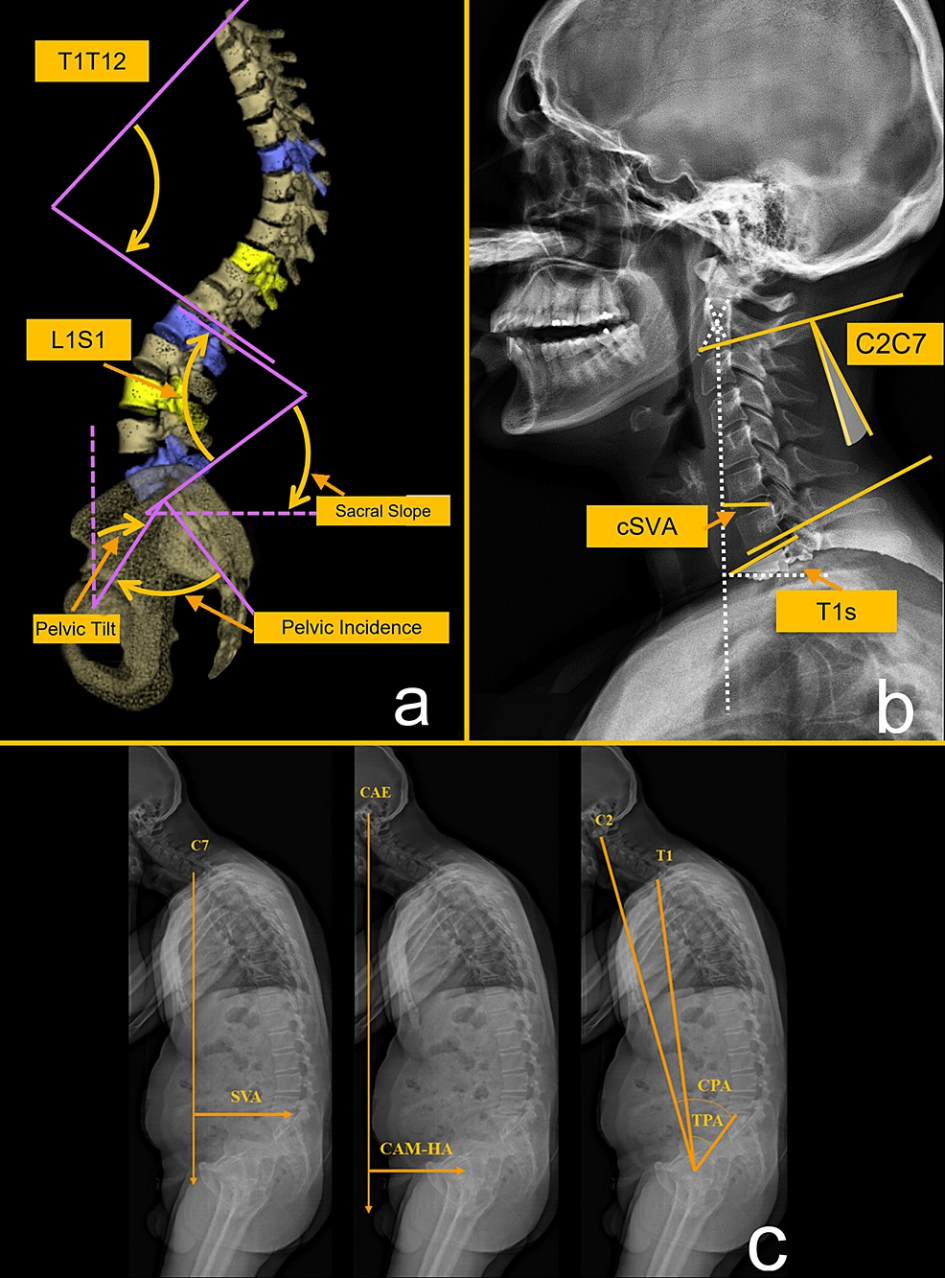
Radiographic parameters: spinopelvic (a), cervical (b), and postural (c). Authors’ own creations T1-T12: TK, L1S1: LL, cSVA: cervical sagittal vertical axis, C2C7: cervical lordosis, T1s: T1 slope, SVA: sagittal vertical axis, CAM-HA: center of the auditory meatus-hip axis, CPA: cervical pelvic angle, TPA: thoracic pelvic angle.
